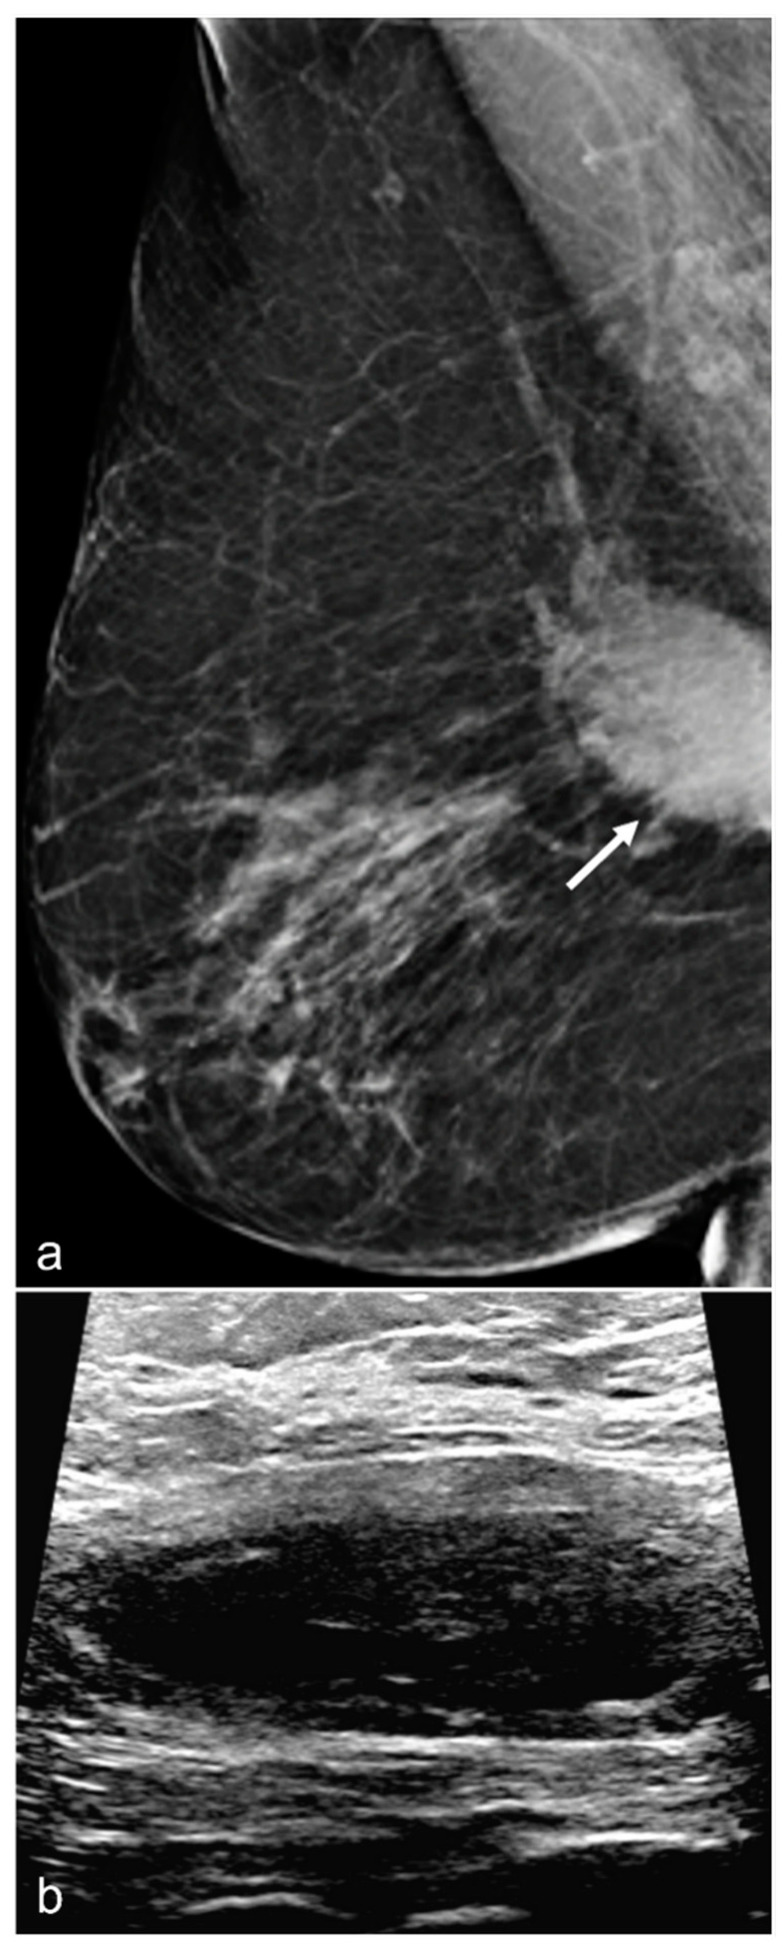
A 67-year-old woman with subglandular silicone implants developed a large, dense right subglandular mass (arrow) on mammography (a) two years after BES. Follow-up longitudinal right breast ultrasound (b) demonstrates a fluid collection with internal debris, confirming evidence of a seroma.
